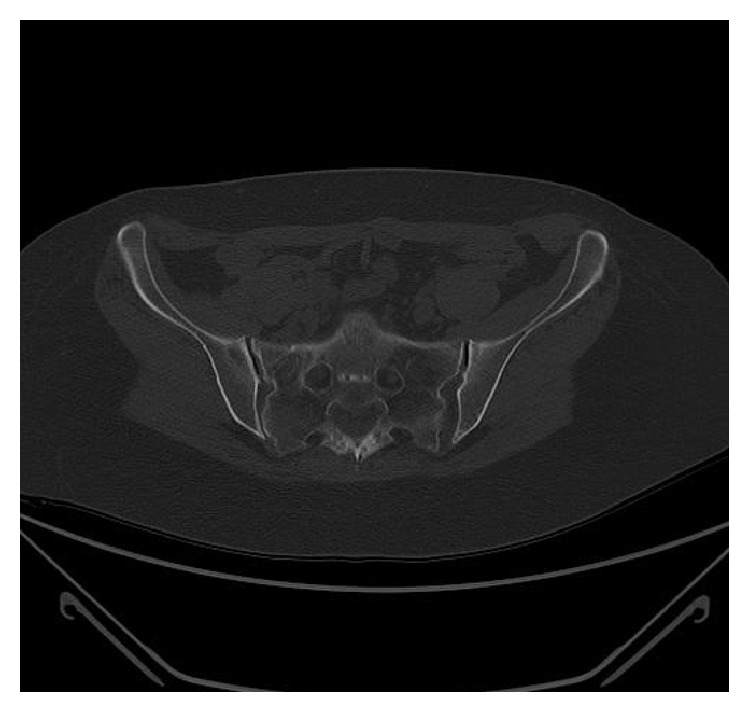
CT scan: axial view that showed the fracture line from the anterior border of sacrum to the right sacroiliac joint.
